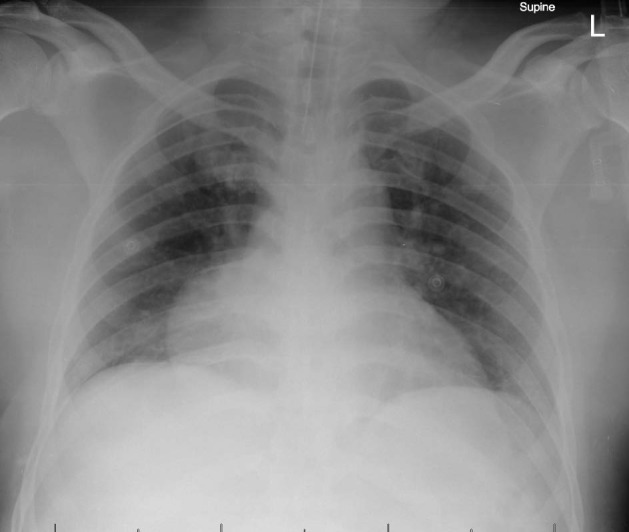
Chest radiograph of patient on arrival in ICU
